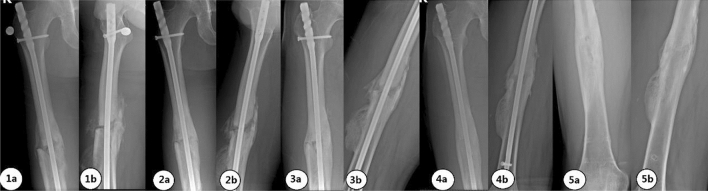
X-ray films at 6 (1), 15 (2), 23 (3), and 35 (4) months after fixation and after removal of the internal fixation (5). Anterior view (a). Lateral view (b).
